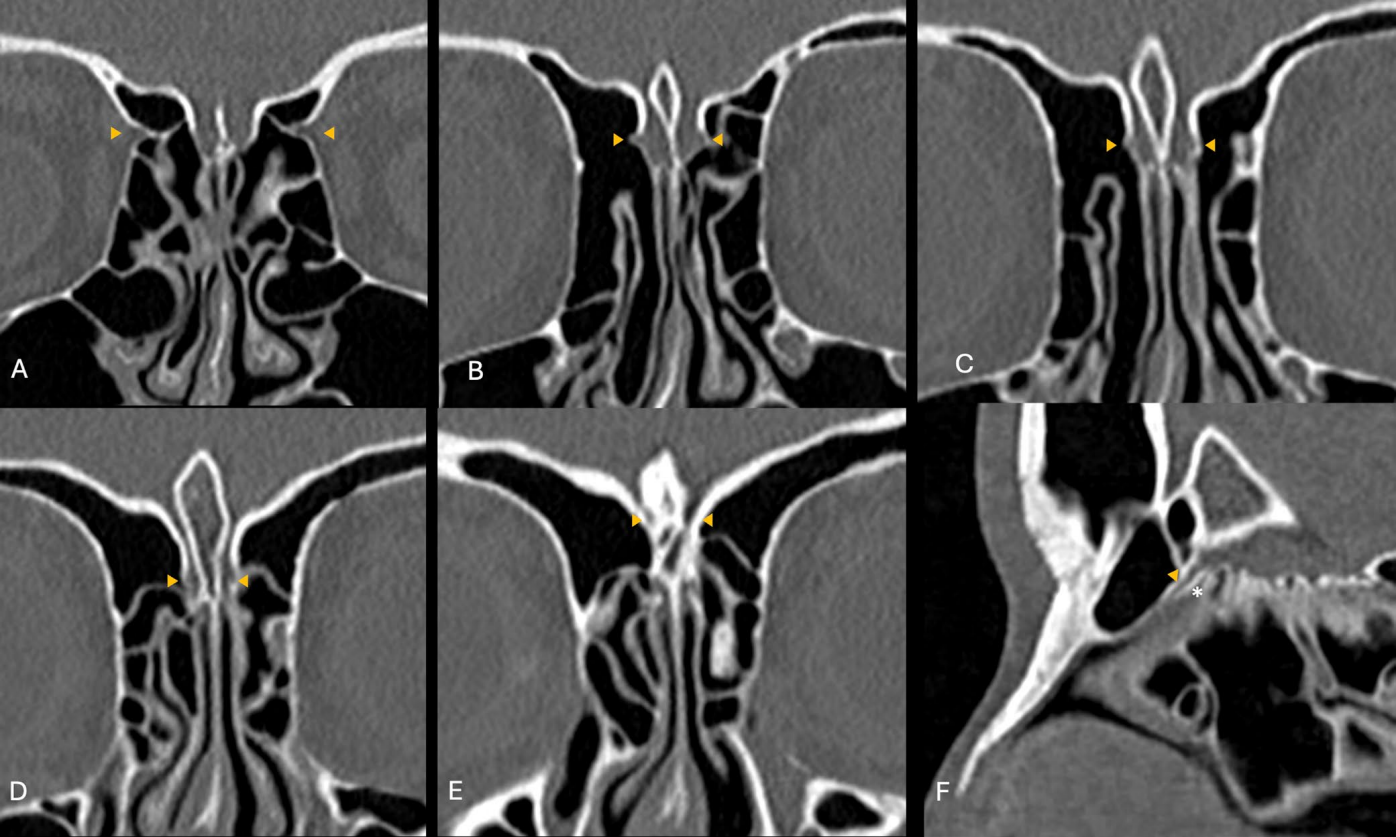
Radiologic analysis of the AEA on CT scans in coronal (A–E) and sagittal (F) planes. Once the AEA enters into the cranial fossa it gives two terminal branches: The anterior meningeal artery and nasal branch. Yellow arrows show the AEA (A–E) and NbAEA (F).
